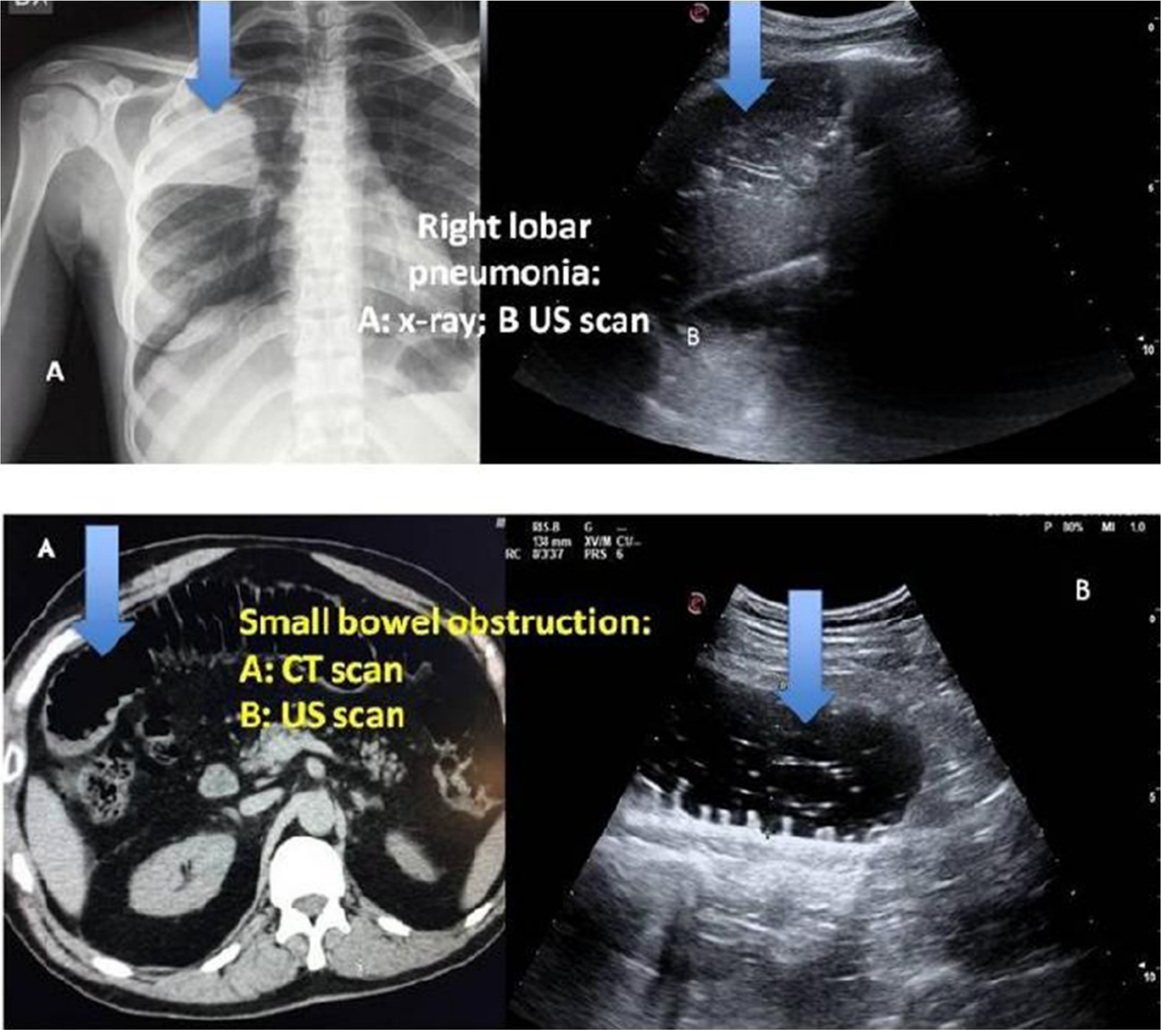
Fig. 1 Top, right lobar pneumonia (A: x-ray; B: US scan). Bottom, small bowel obstruction (A: CT scan, B : US scan)
